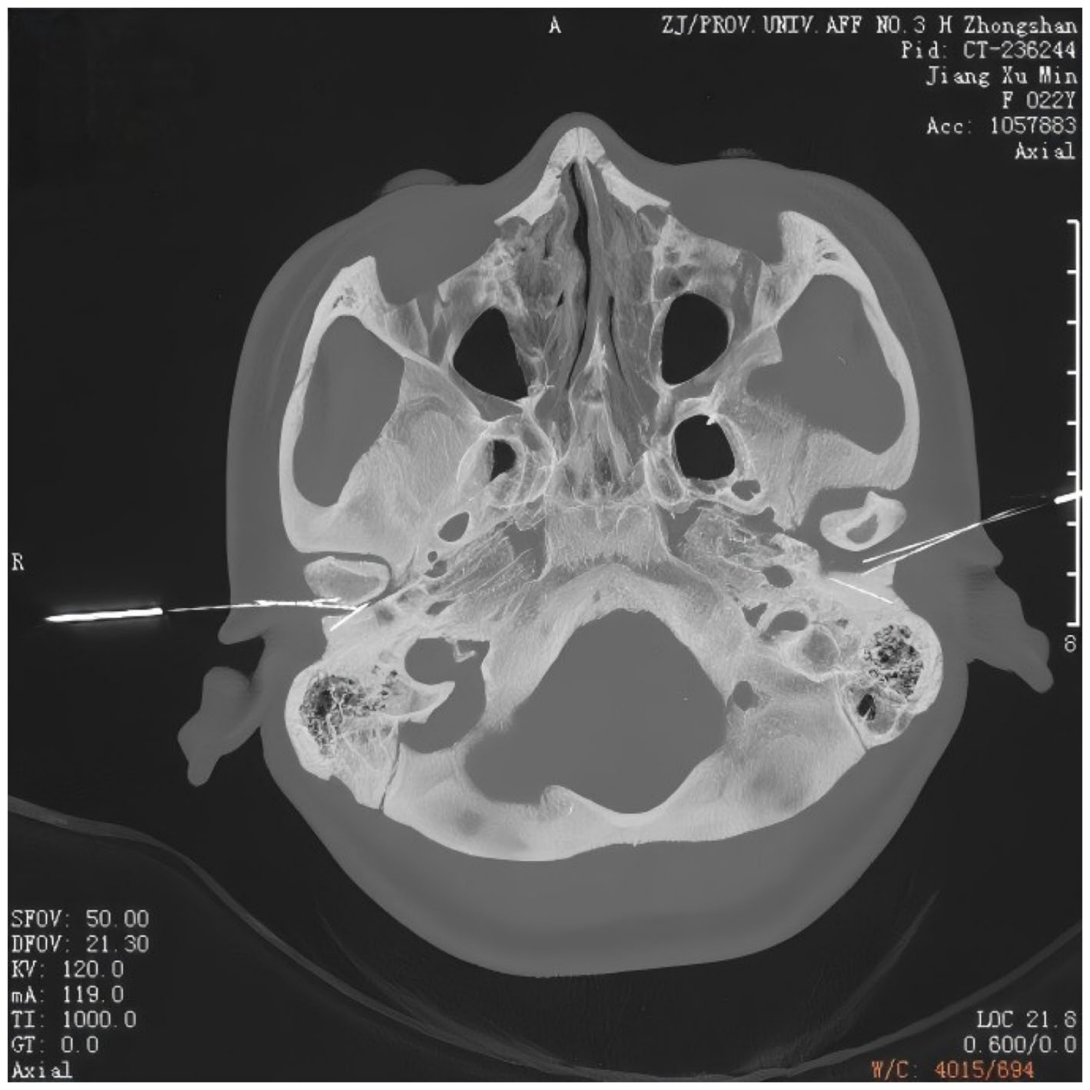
Needling depth CT image.
